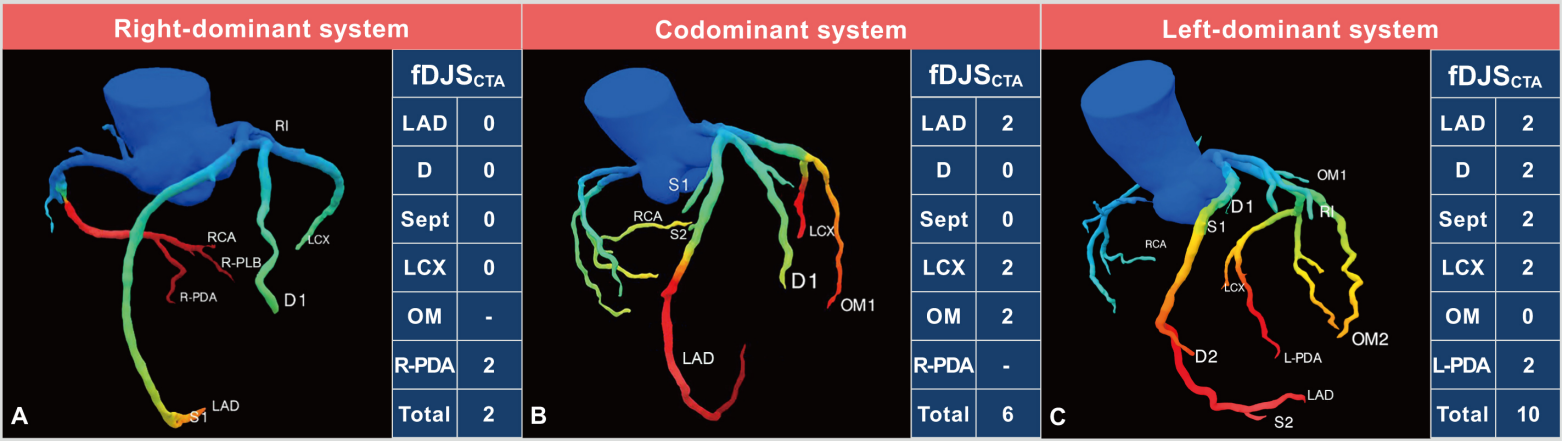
CT-FFR based functional Duke Jeopardy Score system. (A) Right
